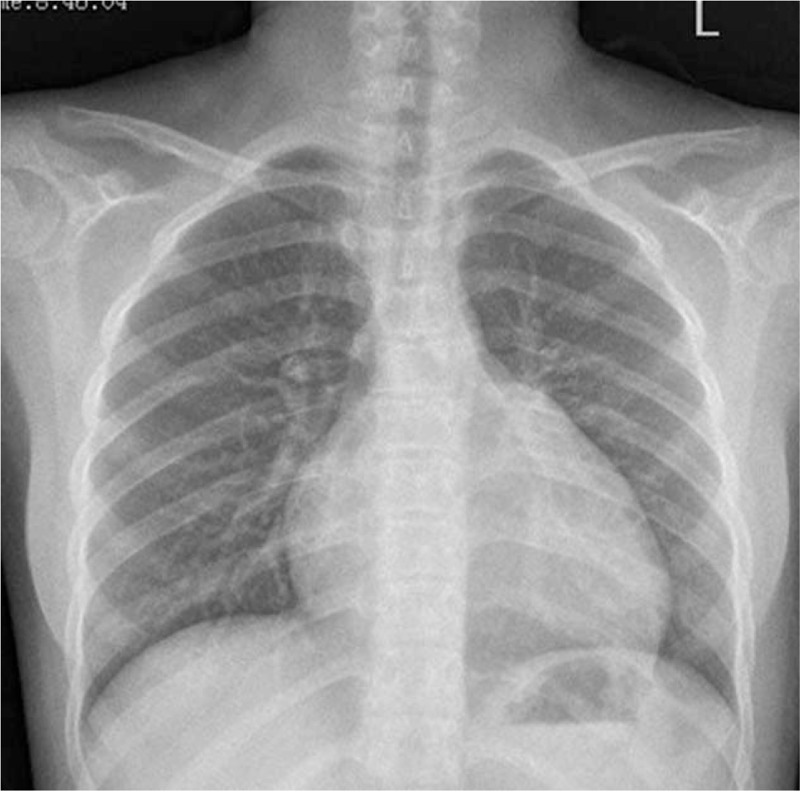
Chest radiograph showed enlargment of cardiac shadow and the cardiothoracic ratio was 0.6.
